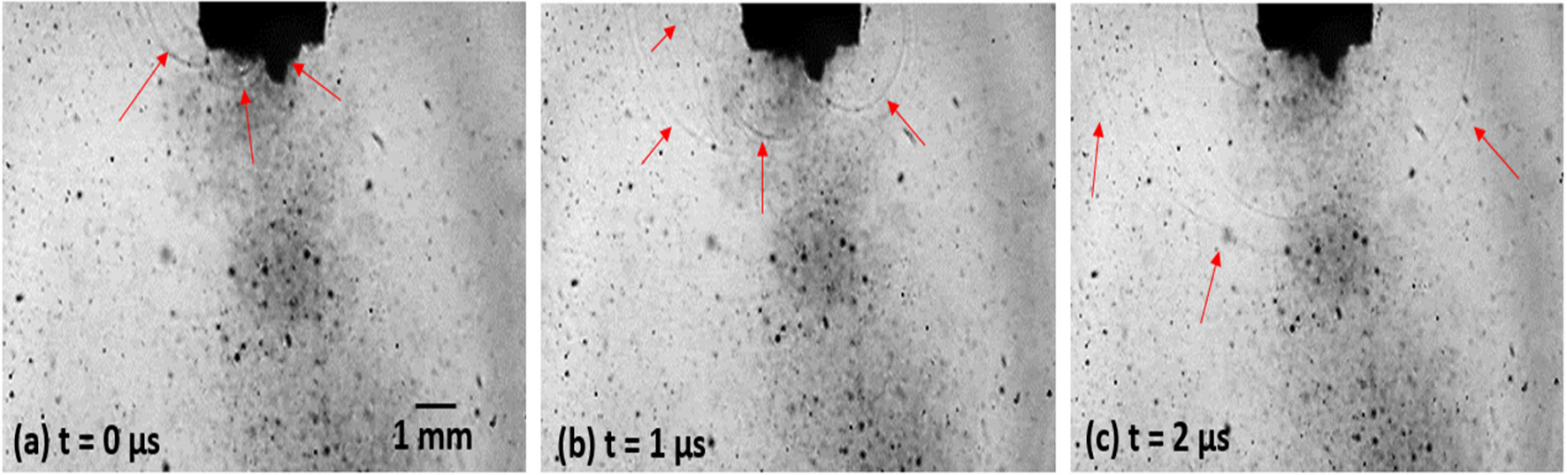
Images of ultrasonic cavitation and shock wave propagation generated from a 3 mm sonotrode recorded at 1Mfps. The supplementary video can be accessed with the online version of the article as video 2.
